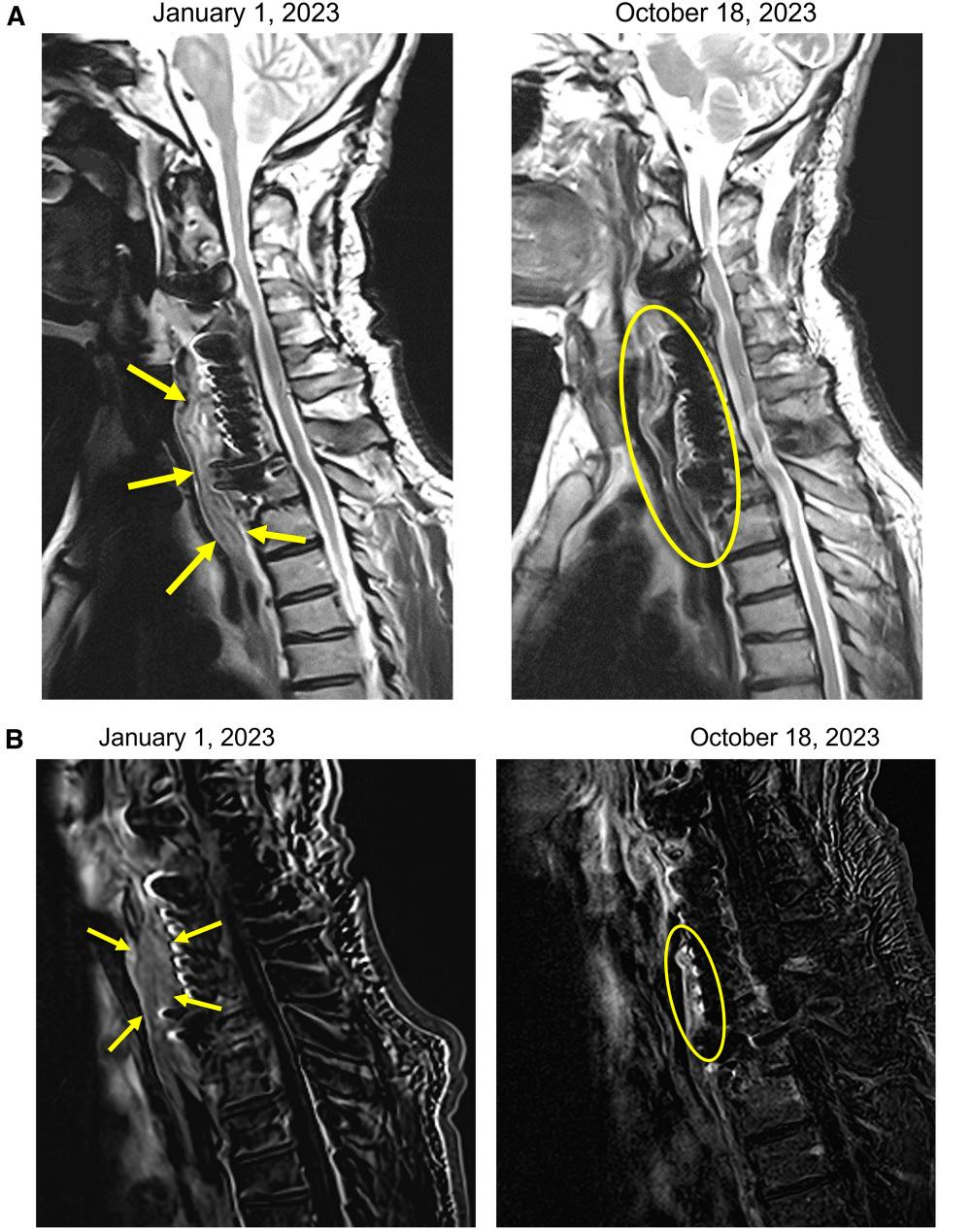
Computed tomography scan images for Case 6 at diagnosis and at 8 months after starting treatment with echinocandin including rezafungin, showing regression of prevertebral soft tissue swelling. The patient required lifelong antifungal therapy and continued rezafungin treatment after the early access program period (ongoing at 19 months). *A*, T2 sagittal image of the cervical spine. *B*, Contrast media subtraction images.
